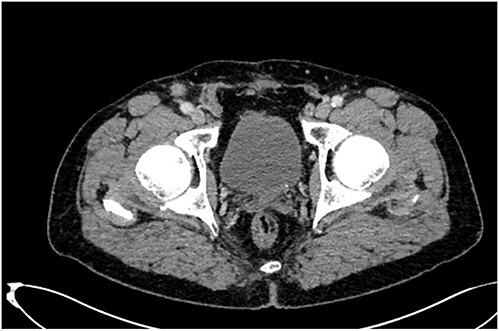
A right inguinal lymph node in a CT scan.
